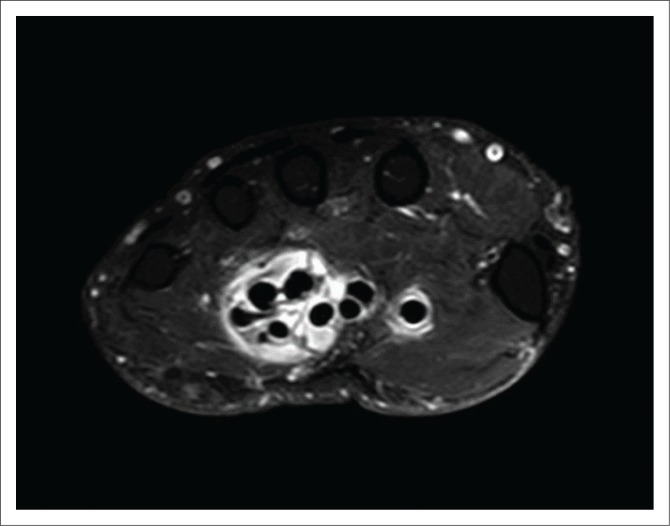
Axial proton density-weighted fat-saturated post-contrast magnetic resonance imaging at the level of the metacarpal bones demonstrating enhancement of the flexor tendons within the flexor compartment in keeping with tenosynovitis.
